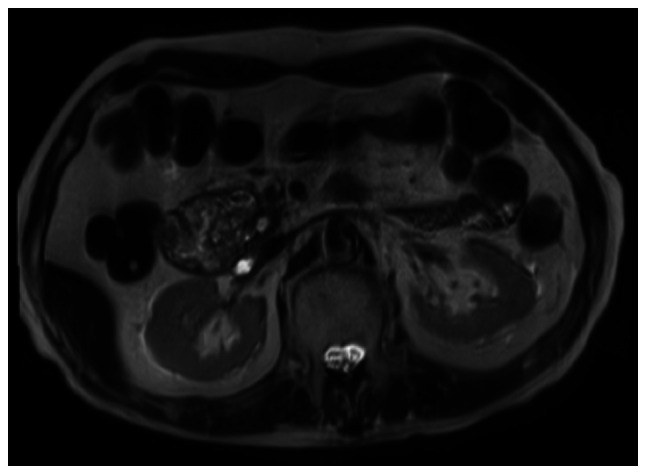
Magnetic resonance cholangiopancreatography signal from a T2-weighted image at the patient's first visit. The pancreatic head tumor exhibited low intensity, with a small high-intensity area.
